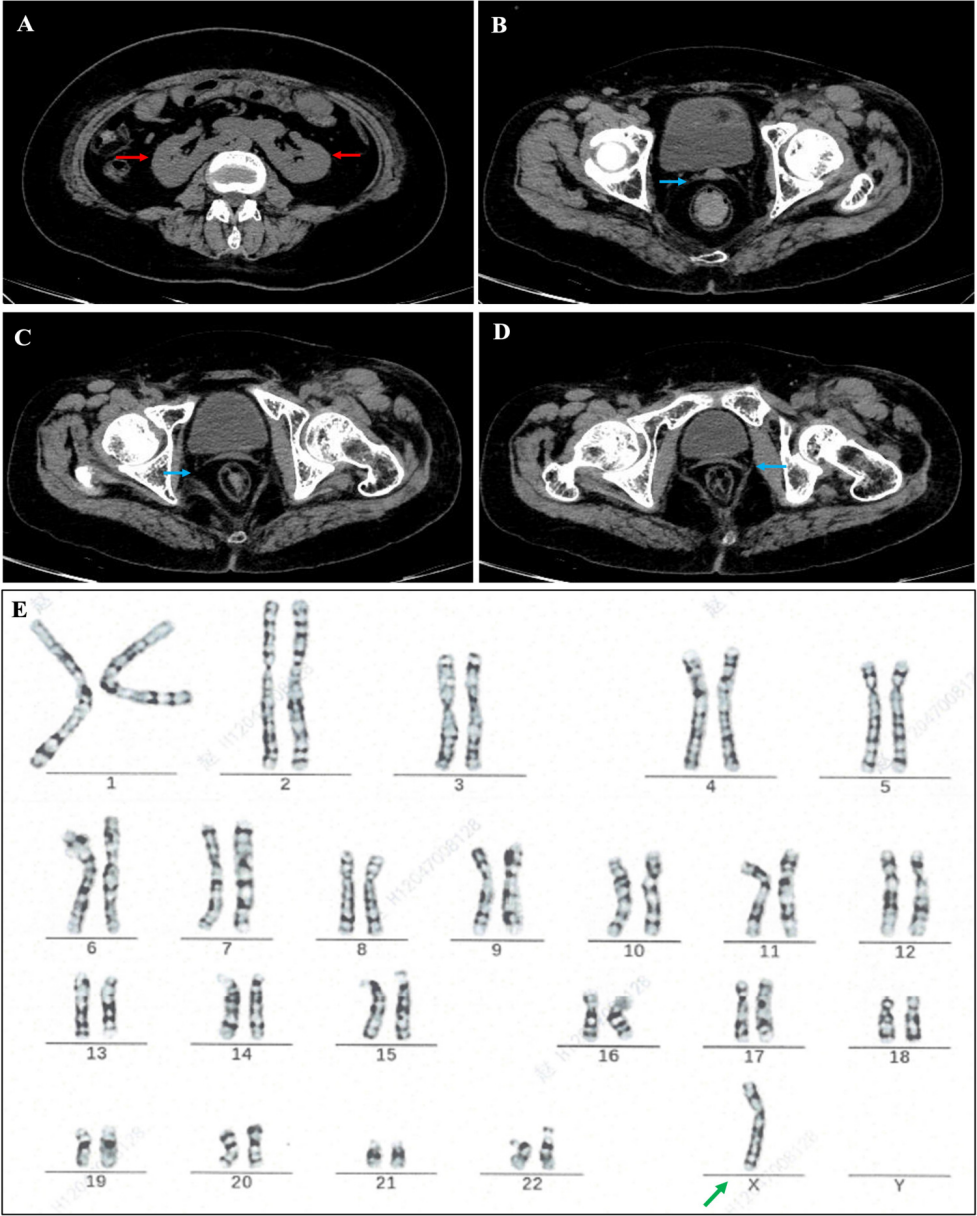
Abdominal computed tomography (CT) showed horseshoe kidney and fused kidney [(A), red arrow], no ovarian structure was seen, and cord-like shadow of uterus was visible [(B–D), blue arrow]; cytogenetic report showing a gene karyotype of 45.X [(E), green arrow].
